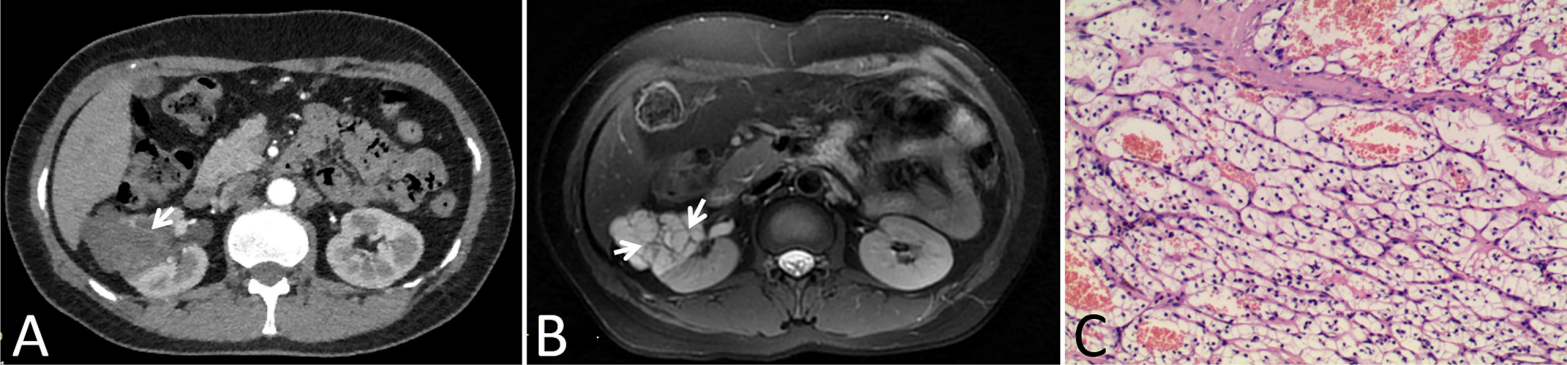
Images in a 46-year-old woman with a cystic mass in the right kidney. (A) Axial contrast-enhanced CT image shown a complex cystic mass that contained a few thin septa (arrow), (B) Axial T2-weighted MR image shown more septa (arrows) within the lesion than were depicted on the CT image, (C) This lesion was surgically removed and determined to be a renal clear cell carcinoma with cystic changes and glassy changes.
